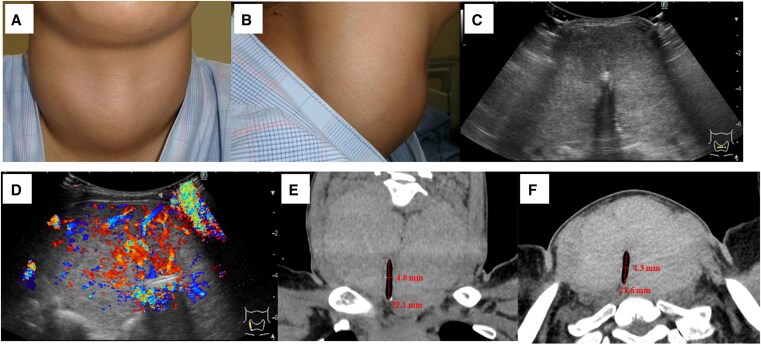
Imaging of the massive goiter. (A, B) Physical examination revealed a large goiter of WHO Classification Grade III with no tenderness or palpable mass. (C, D) Ultrasonography revealed marked thyroid enlargement with diffuse hypervascularity. The right lobe measured 5.7 × 5.2 × 8.8 cm and the left lobe 5.2 × 4.9 × 10.0 cm (anterior–posterior × transverse × longitudinal). No hypoechoic areas or masses were observed. (E-F): CT scan showing goiter (volume: 366 mL) associated with tracheal compression and narrowing with a minimal diameter of 4.3 mm.
